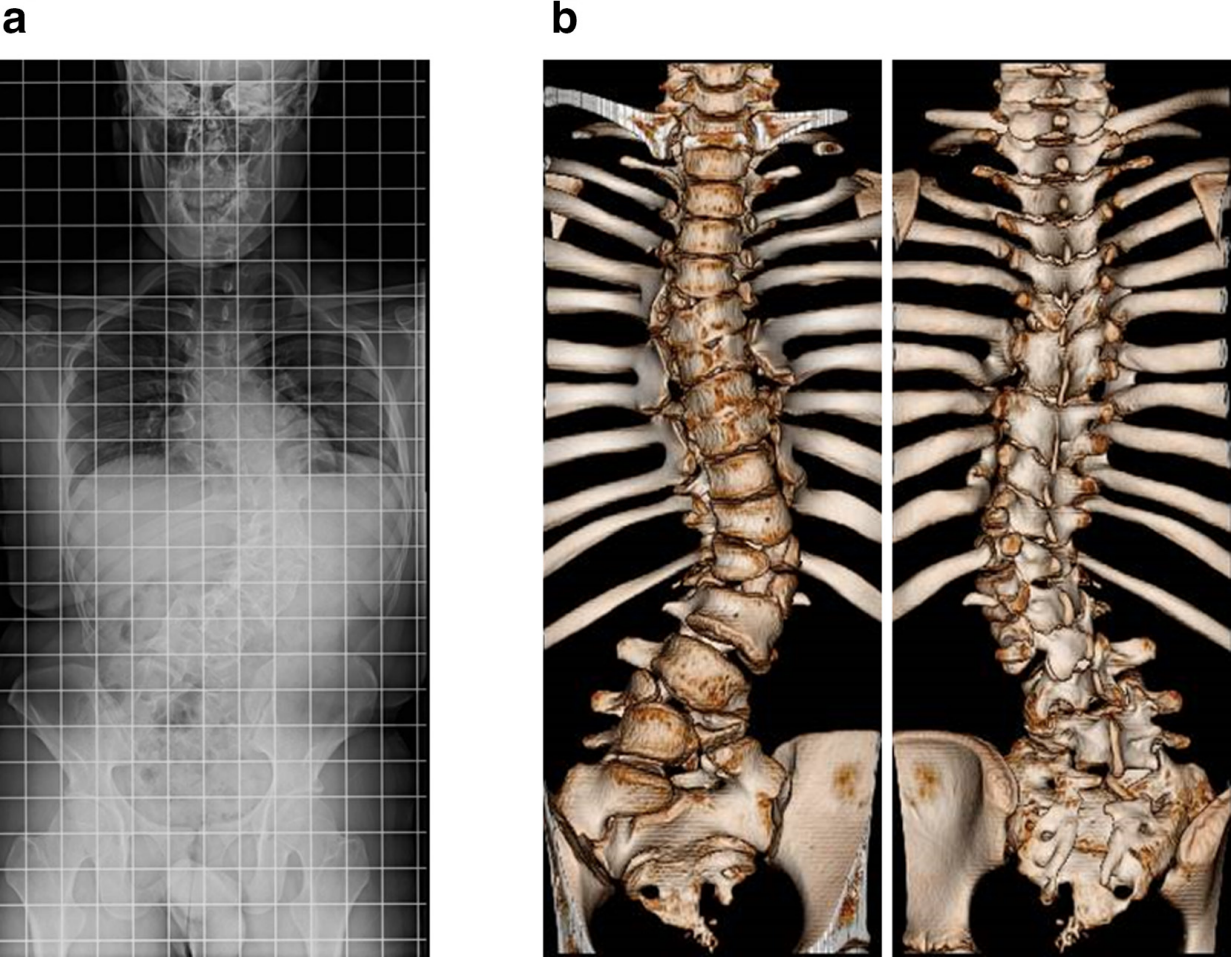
Radiographic phenotype of the congenital scoliosis patient with *LFNG* mutations. (a) The standing spinal antero‐posterior radiograph. The patient has severe scoliosis with a Cobb angle of 70° at T9‐L3. (b) Three‐dimensional CT (left: front view, right: back view). Multiple congenital vertebral malformations including hemivertebrae, block vertebrae, and butterfly vertebrae are noted. Multiple rib malformations including hypoplasia and fused ribs are also found
